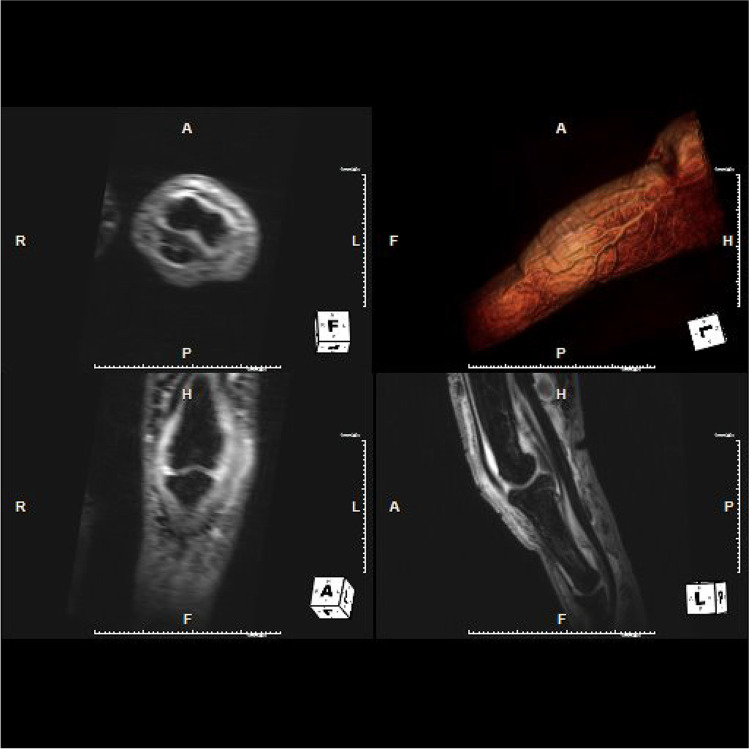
MPR reconstruction on 3 planes of 3D high-resolution T1-weighted with fat saturation of the second right finger of a 43-year-old man. There is distension and enhancement of capsule/synovium of the proximal interphalangeal joint. There is flexor and extensor tenosynovitis and enhancement of their synovial sheaths without enhancement at the enthesis
